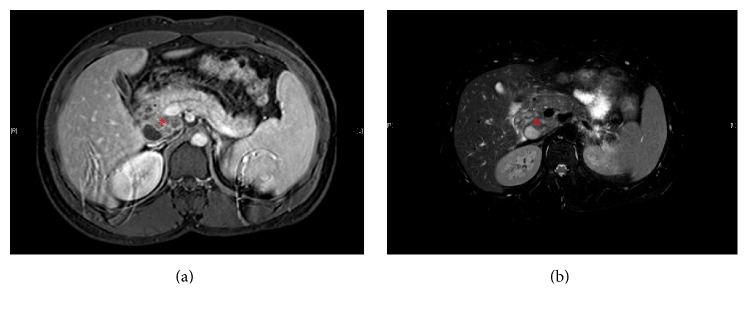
Axial abdominal MRI. (a) T1 MRI with contrast in arterial phase and (b) T2 MRI. Demonstrating a multiloculated complex cystic mass at the posterior aspect of the pancreatic head with heterogeneous content and enhancing septations (asterisk). It measures 4.7 × 4 cm with mild mass effect on the portal vein and no definite communication with common bile duct or pancreatic duct.
